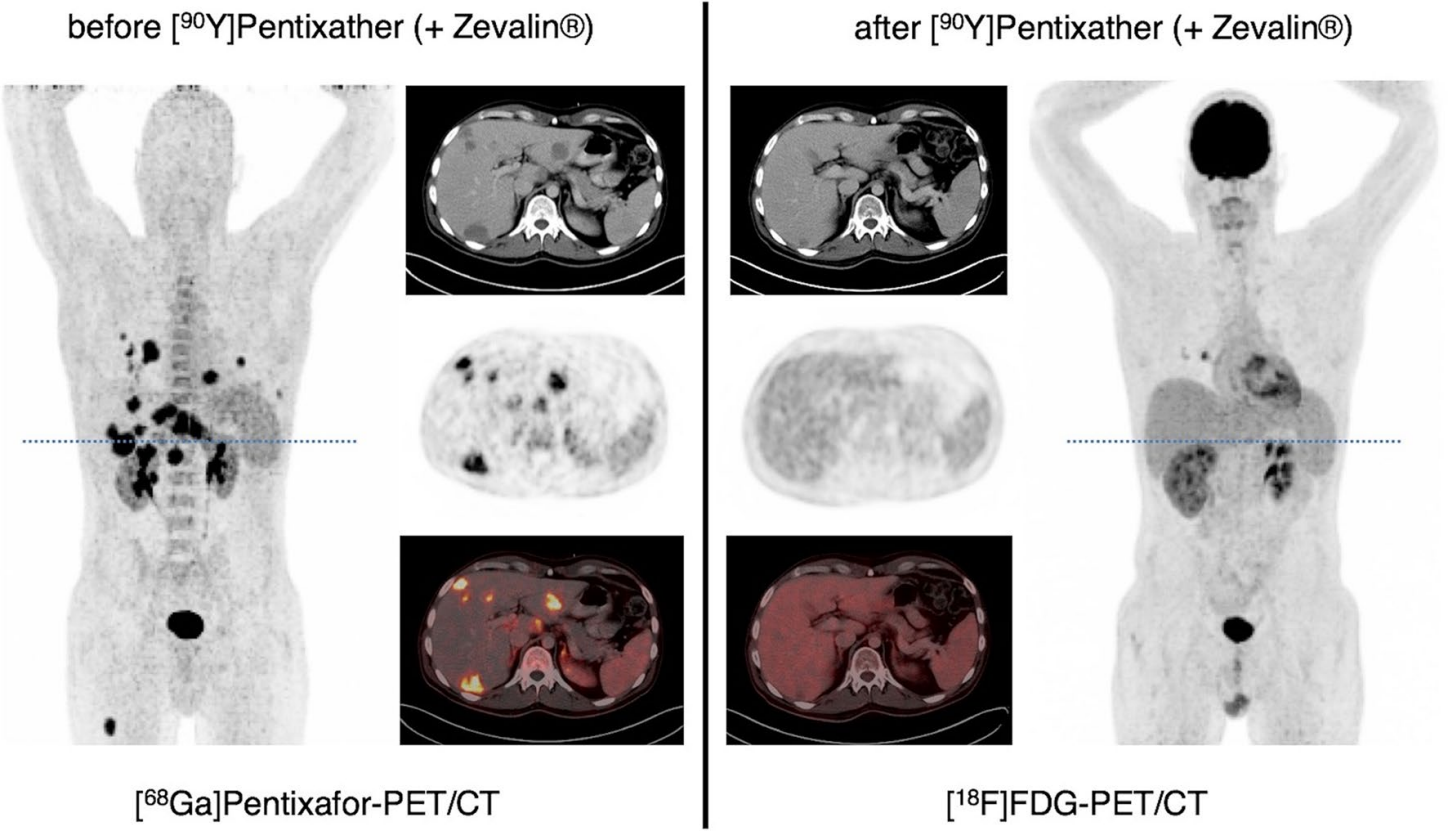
Example of CXCR4-directed endoradiotherapy with [^90^Y]Pentixather (in combination with CD20-directed radioimmunotherapy with [^90^Y]Zevalin^®^) as part of the conditioning regimen prior to allogeneic stem cell transplantation in relapsed/refractory diffuse large B cell lymphoma (DLBCL). Display of maximum intensity projections (outer columns) and transaxial slices (inner columns; CT, upper row, PET, middle row; PET/CT, lower row) of pre-therapeutic CXCR4-directed and post-therapeutic [^18^F]FDG PET/CT. Post-therapeutic imaging was performed 8 weeks after administration of 3.7 GBq of [^90^Y]Pentixather, 1.2 GBq of [^90^Y]Zevalin^®^ as well as conditioning chemotherapy with subsequent repeat stem cell transplantation (SCT) and demonstrated partial response with residual yet vital pulmonary lesions and resolution of all hepatic and nodal DLBCL manifestations. DLBCL had been relapsed from prior first allogeneic SCT and been refractory to all chemotherapeutic regimens
